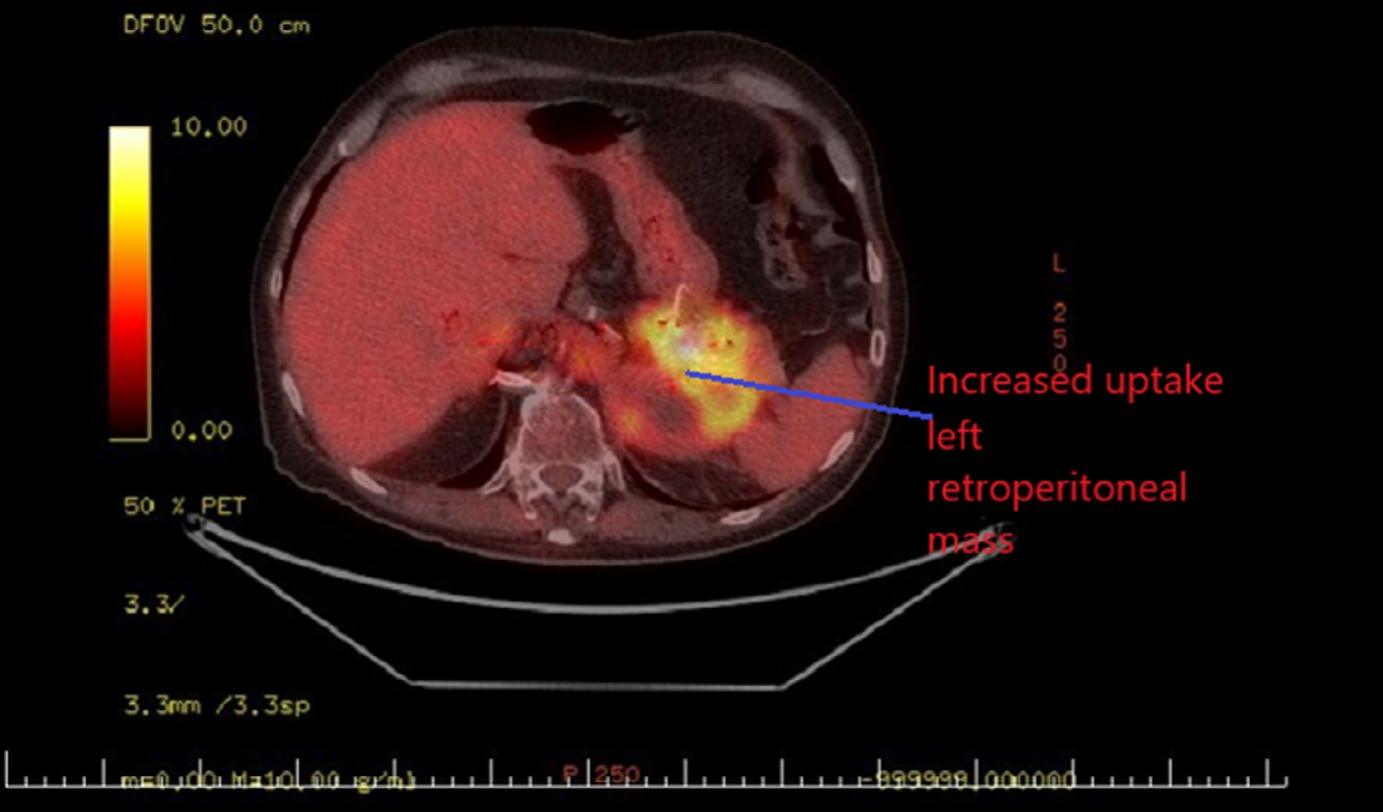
PET/CT scan showing a left adrenal tumor with SUV_MAX_ uptake:18
